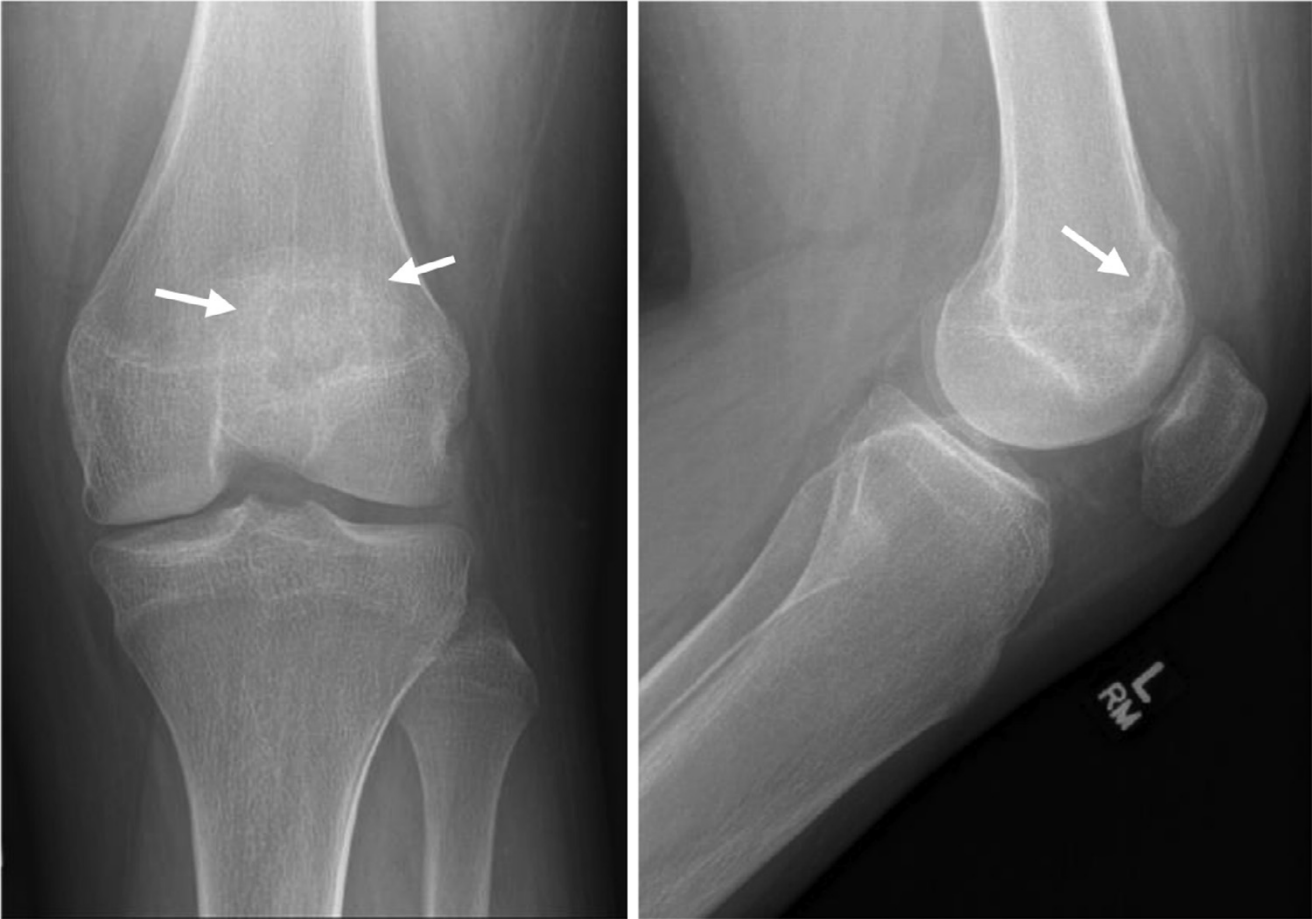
AP and lateral radiographs of the knee showing a very subtle radiolucent lesion in the distal femoral epiphysis involving the physis and metaphysis. The lesion has faint sclerotic margin (white arrows)
